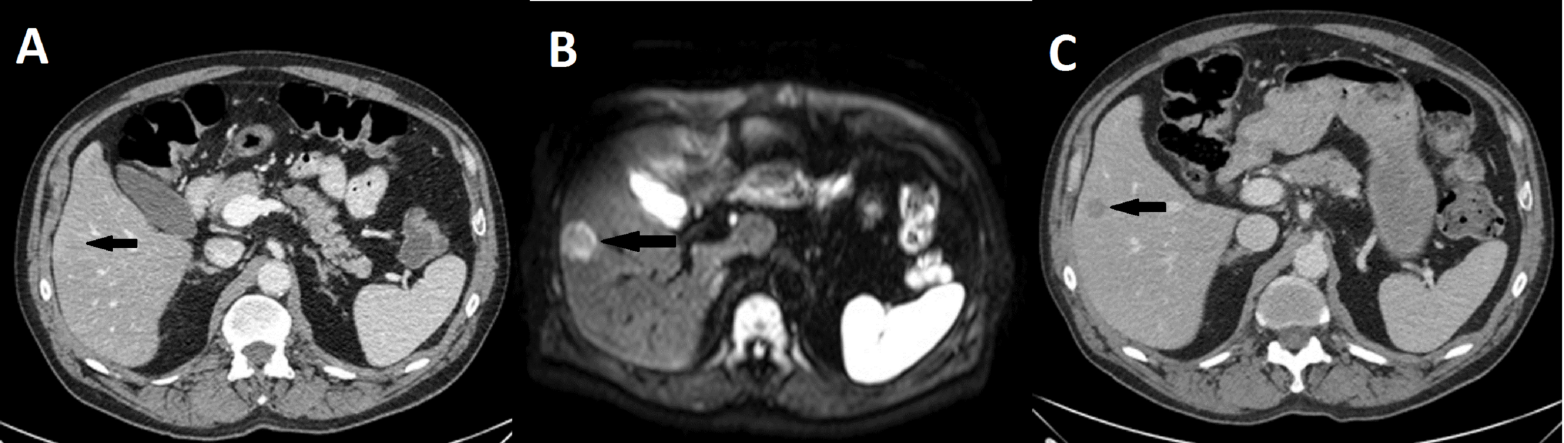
Missed metastasis on initial CT and diffusion weighted MR. (A) Initial CT, (B) Diffusion weighted MR, (C) CT in venous phase after 3 months of neoadjuvant therapy. Black arrow: Metastasis missed on primary CT.
